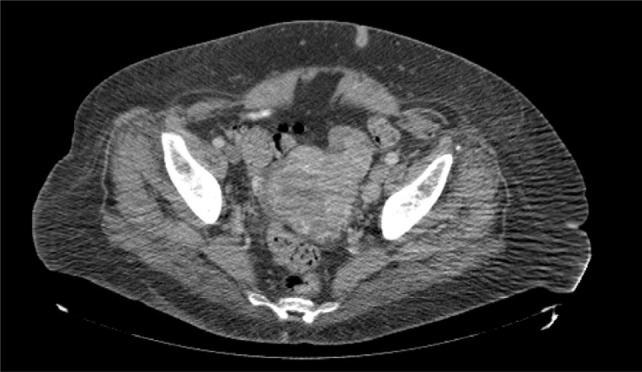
Axial view of computed tomography of the abdomen and the pelvis revealing a heterogeneous appearance of the uterus.
